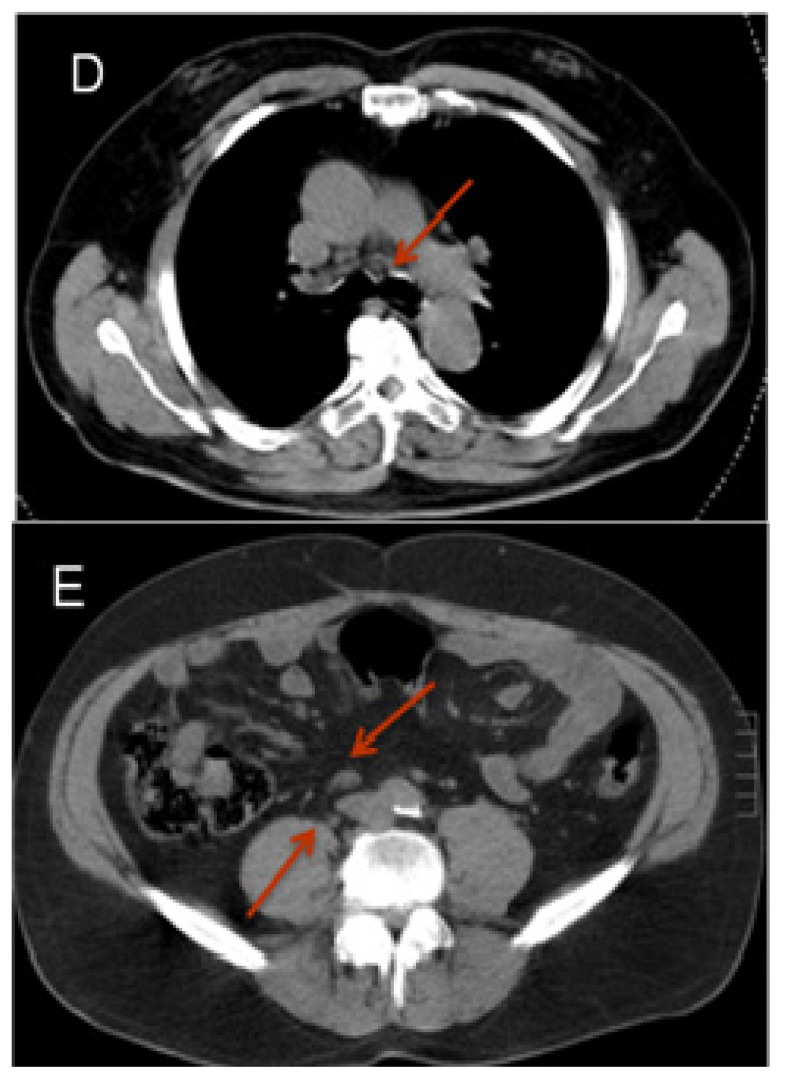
^68^*Ga-PSMA-PET-CT. Patient with prostate cancer (status after brachy-therapy and bilateral iliac lymph node dissection, current PSA 21 μg/L). PET images were acquired after the administration of *^68^*GA-PSMA-Ligand (60 min thereafter). The figure shows fused images (PET-CT): On the coronal view (A) a pathologic isotope uptake in multiple lymph nodes in the right para-iliac region and infra-carinal (mediastinum) is clearly visible; the corresponding transversal images show the para-iliac (B) and infra-carinal (C) lymph nodes with elevated uptake of the tracer. The corresponding transversal native CT images present these suspect structures (D,E) as normal sized lymph nodes (marked by red arrows).
